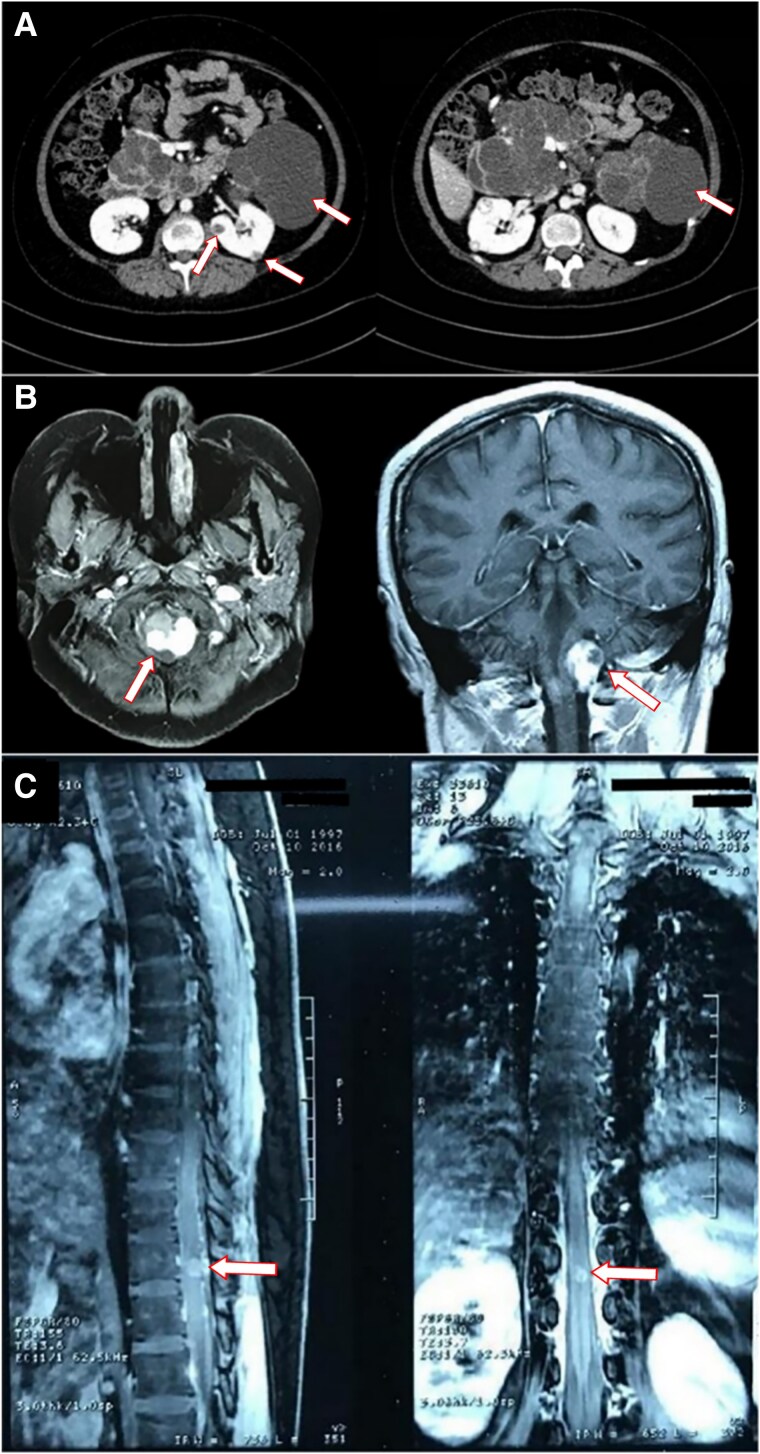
Some results of imaging examination. A, The abdominal enhanced computerized tomography (CT). The arrows show the cysts in both kidneys and pancreas. B, The magnetic resonance imaging (MRI) of the head. The arrows show the lesion in bilateral cerebellar bulbar cisterna. C, The magnetic resonance imaging (MRI) of the thoracic vertebrae. The arrows show the lesion in the thoracic vertebrae.
